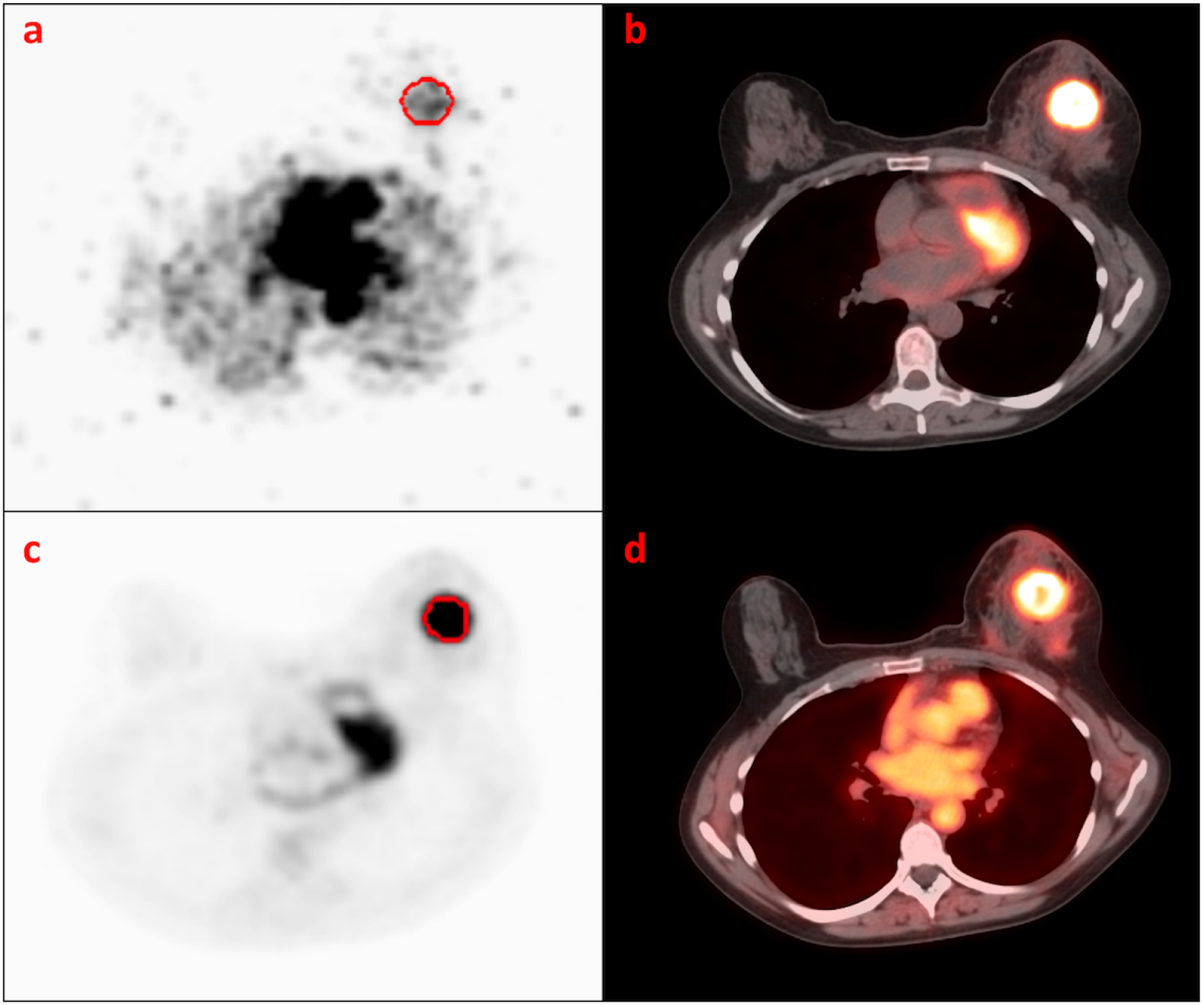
PET images of a 54-year-old woman with a T2-N1-M0 TN (HR-negative, HER2 non-amplified) ductal carcinoma. a parametric blood flow (mL/min/g) image from ^18^F-FDG PET first-pass dynamic (10-s frames) acquisition, b fusion of the first 8-min list-mode ^18^F-FDG PET and the associated CT, from which the dynamic first-pass image is reconstructed, c SUV parametric image from delayed (90 min post injection, 4-min per bed position) acquisition, d fusion of the delayed PET aquisition (Bq/mL) and the associated CT. The tumour was manually delineated on the blood flow image (a) and using a semi-automatic algorithm based on a contrast-dependent method [33] on the SUV parametric image (c)
